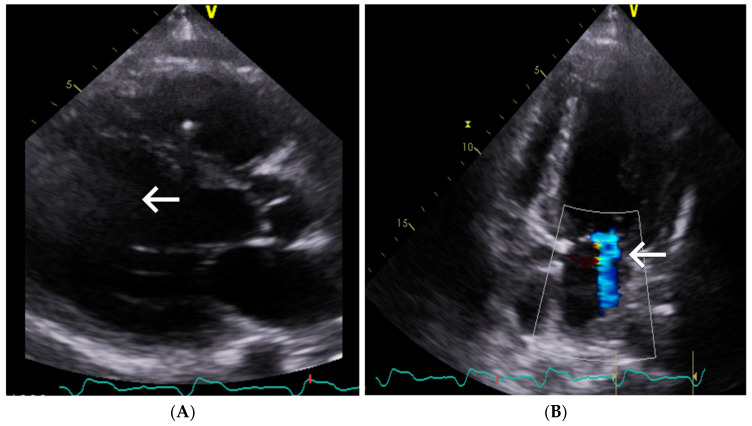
Transthoracic echocardiography. (A) Dilated cardiomyopathy with severe left ventricular dysfunction (LVEDD 68 mm, LVEF 30%) and right ventricular enlargement. (B) Hemodynamically significant mitral regurgitation visualized on color Doppler. White arrows indicate the key pathological findings in each panel, and color Doppler signals denote the regurgitant jet.
